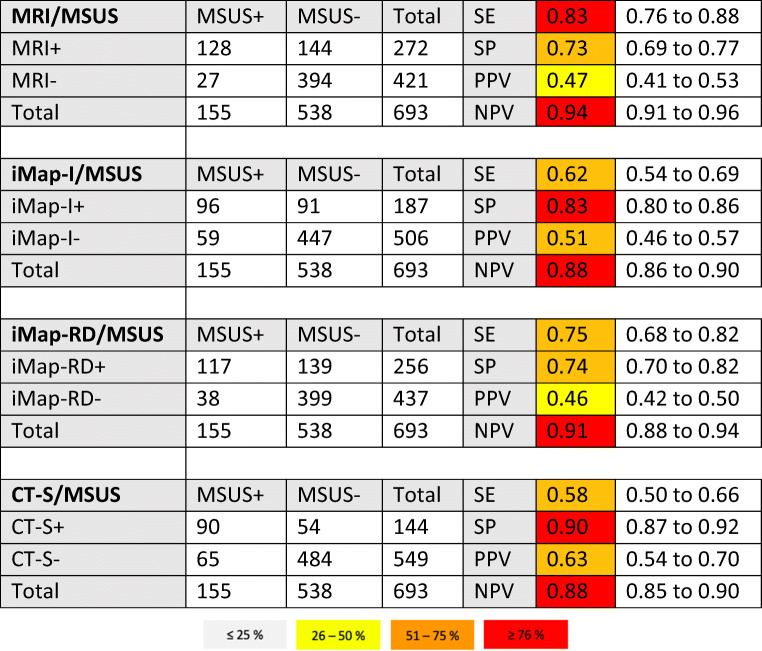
Results of contingency table analysis with 95% CI of the (modified) RAMRIS synovitis score. *MSUS* musculoskeletal ultrasound, *DECT* dual-energy CT, *iMap-I* image-based iodine map DECT, *iMap-RD* raw data-based iodine map DECT, *CT-S* CT subtraction, *MRI* magnetic resonance imaging, *SE* sensitivity, *SP* specificity, *PPV* positive predictive value, *NPV* negative predictive value
